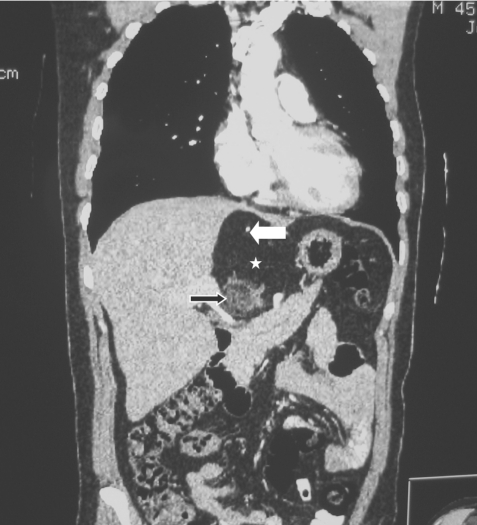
Coronal CT scan of the abdomen. The mass showed combination of fat (asterisk), calcification (white arrow) and soft tissue (black arrow).
